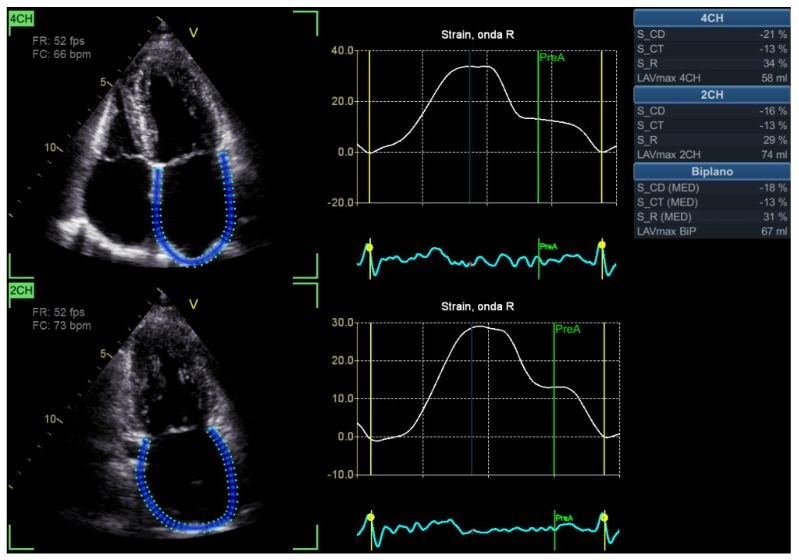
An example of LA function evaluation by LA strain technique, assessed from 2D apical four-chamber (above) and two-chamber (below) views. In light of current recommendations, particularly, biplane LARS represents an important diastolic parameter. Note: 2D, two-dimensional; LA, left atrium/atrial; LARS, LA reservoir strain (peak atrial longitudinal strain) (see text, tables, and references).
